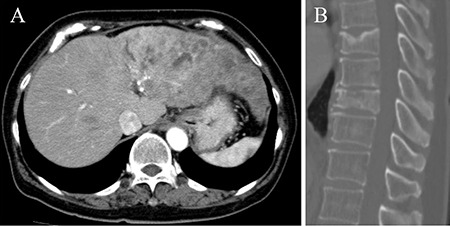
A, B. Axial contrast-enhanced computed tomography of the abdomen showing multiple liver masses (A), and sagittal contrast-enhanced computed tomography of the abdomen showing vertebral bodies metastases (B).
